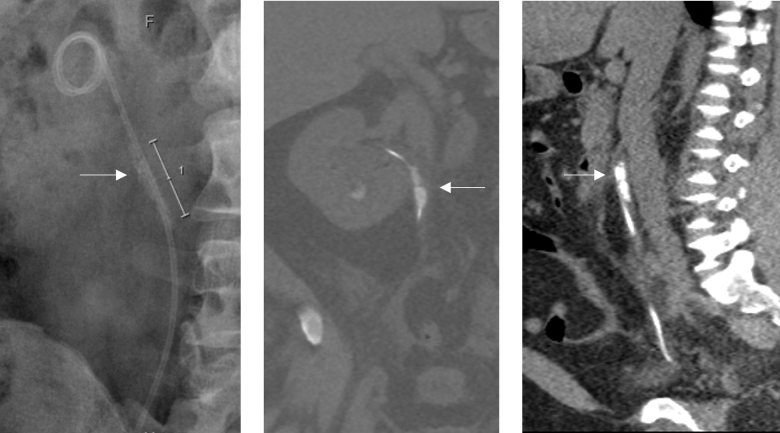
KUB radiograph and CT KUB imaging showing encrustations (indicated by *arrow*) in the upper part of the ureteral stent. KUB, kidney, ureter, and bladder.
